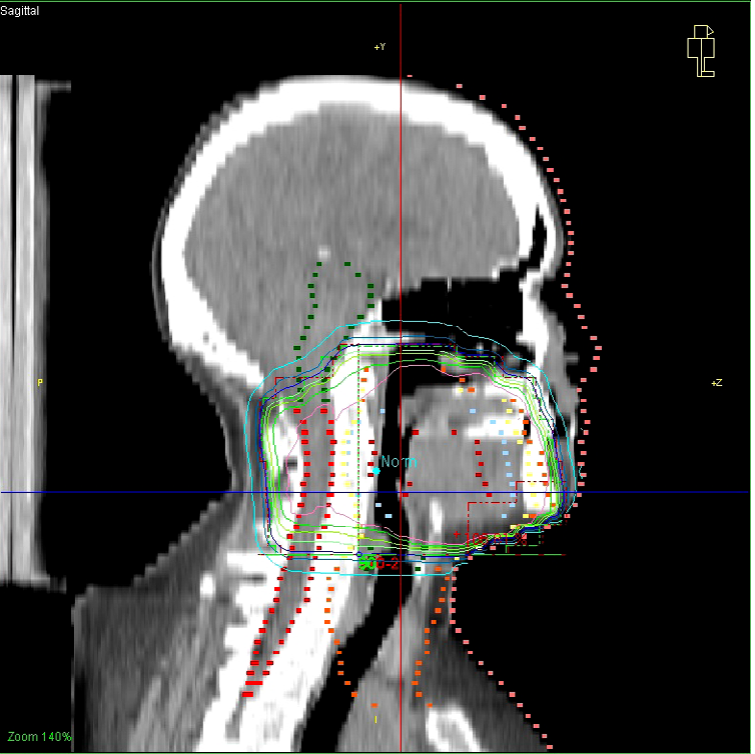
Corresponding digitally reconstructed radiograph of the radiation portals.
